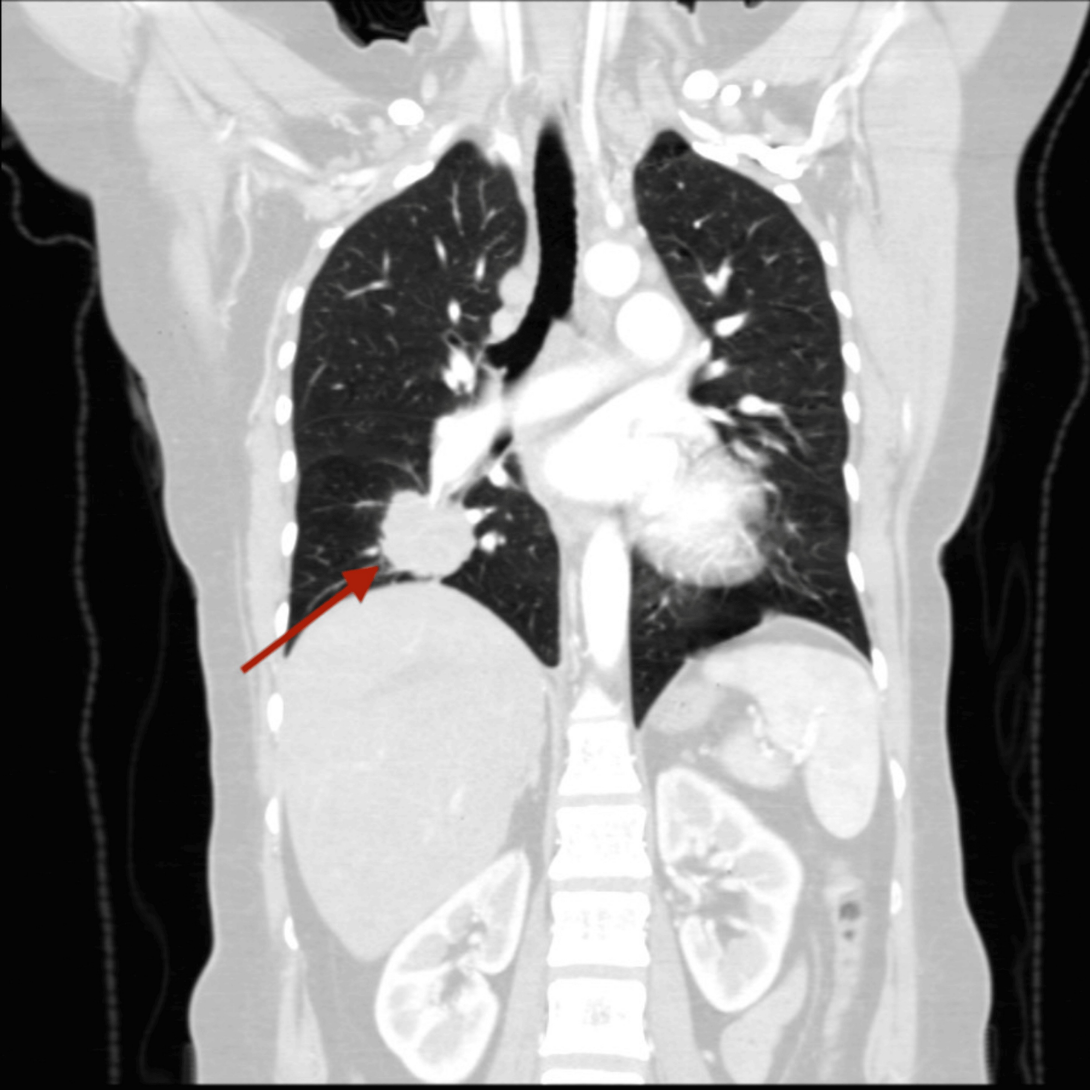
CT chest showed right lower lobe suspicious mass with bronchial obstruction/endobronchial extension, mediastinal lymphadenopathy
